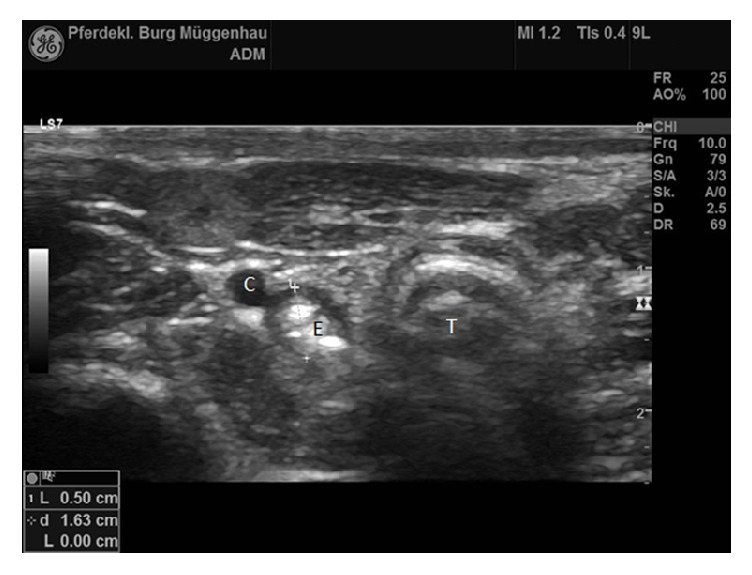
Ultrasound image of the stricture site. Left in the image is dorsal. A substantial decrease in diameter compared to being immediately cranial to the stricture can be observed. C: carotid artery. E: esophagus. T: trachea (probe frequency 10 MHz, depth 2,5 cm).
